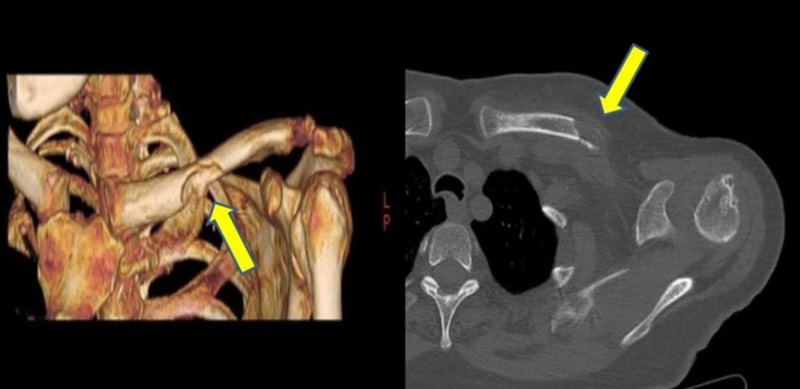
Lytic mass lesion in the middle of the left clavicula and pathologic fracture in the CT image
